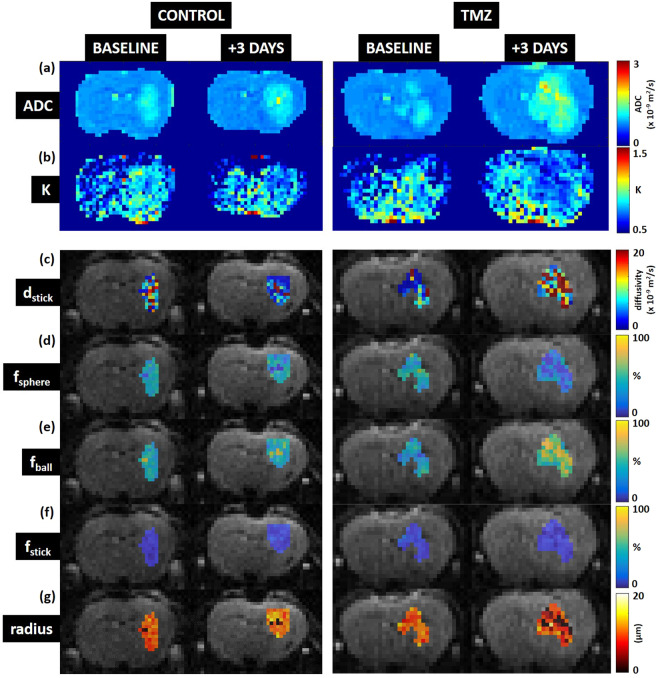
ADC, mean kurtosis and VERDICT model parameter maps at baseline and 3-days post therapy. (a) Apparent diffusion coefficient (ADC), (b) mean kurtosis (K), (c) *d*_*stick*_ (stick diffusivity), (d) *f*_*sphere*_ (sphere fraction), (d) *f*_*ball*_ (ball fraction), (e) *f*_*stick*_ (stick fraction), (f) sphere radius. Note: VERDICT is designed to model cancer tissue and is not a suitable descriptor for normal brain tissue, therefore VERDICT parameter maps are masked by the tumour ROIs drawn on T1-weighted gadolinium images.
